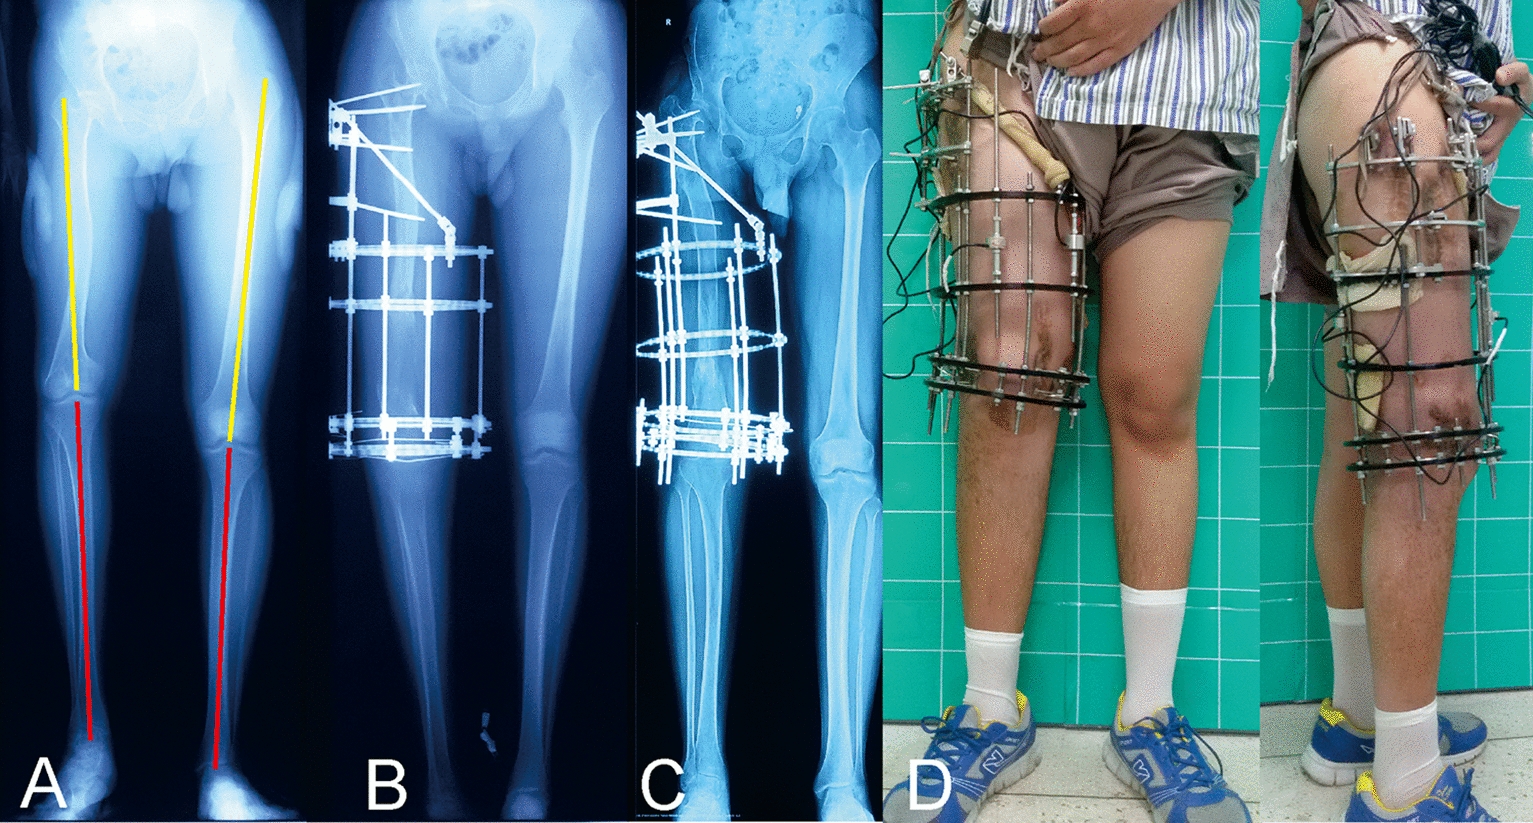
Images of a 20-year-old male patient with limb discrepancy in right femur treated by the circular external fixator using Ilizarov distraction osteogenesis technique. a Initial radiograph manifests the limb discrepancy (9 cm) in the right femur. b Radiograph immediately after the target length is achieved. c Bone lengthening was completed with good regenerate consolidation before the removal of the external fixator. d General appearances during the mechanical test, and the axial load–share ratio is 6.5% at that time
